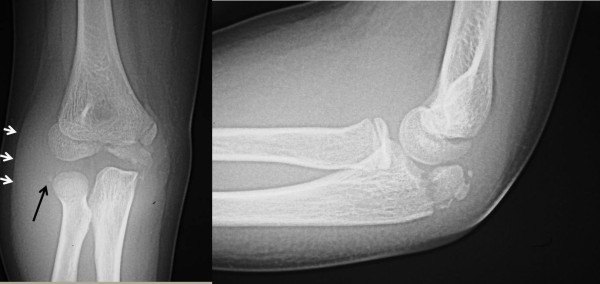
Anteroposterior and lateral radiographs of the elbow. (A) Anteroposterior radiograph showing a small avulsion of the lateral epicondylar fragment (long arrow) and soft-tissue swelling (short arrows). (B) Lateral radiograph showing the olecranon fracture.
